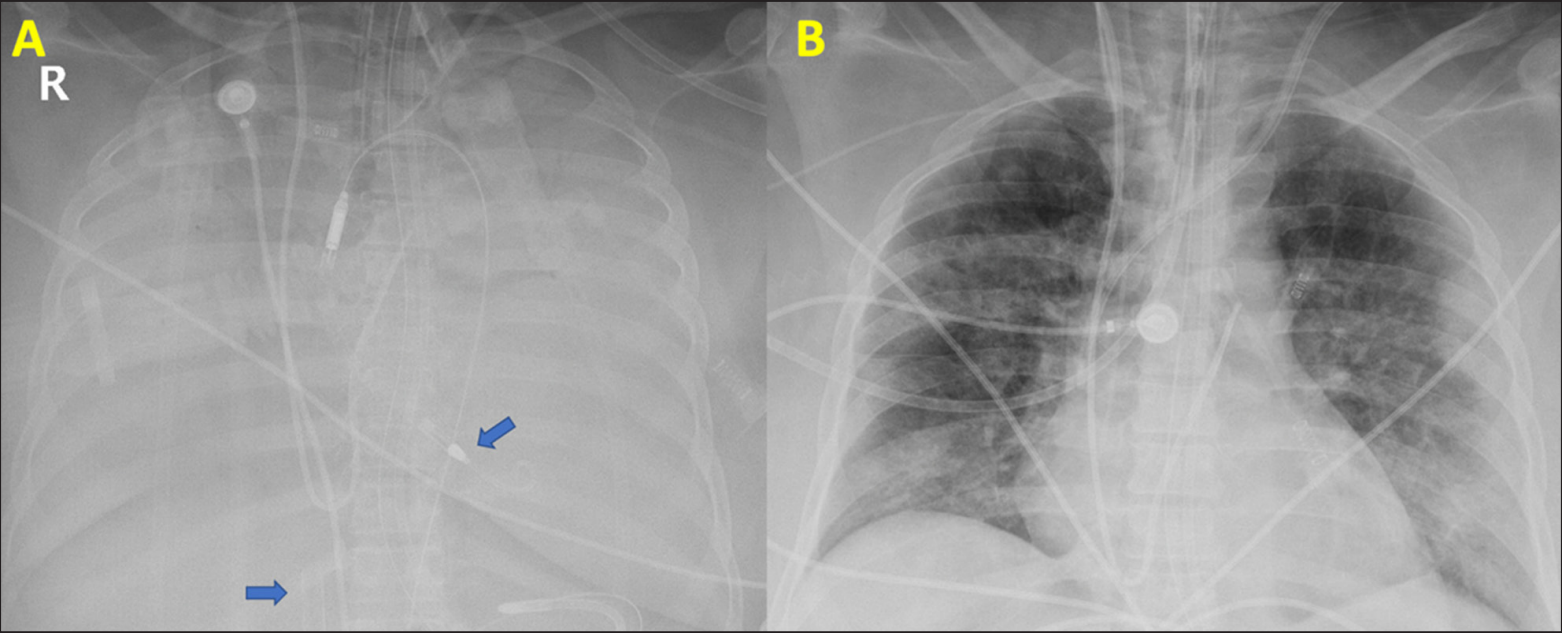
(A) Chest x-ray demonstrating severe COVID-19 pneumonia with extracorporeal membrane oxygenation (ECMO) cannula and Impella (arrows). (B) Post-decannulation chest x-ray after ECMO and Impella removal.
